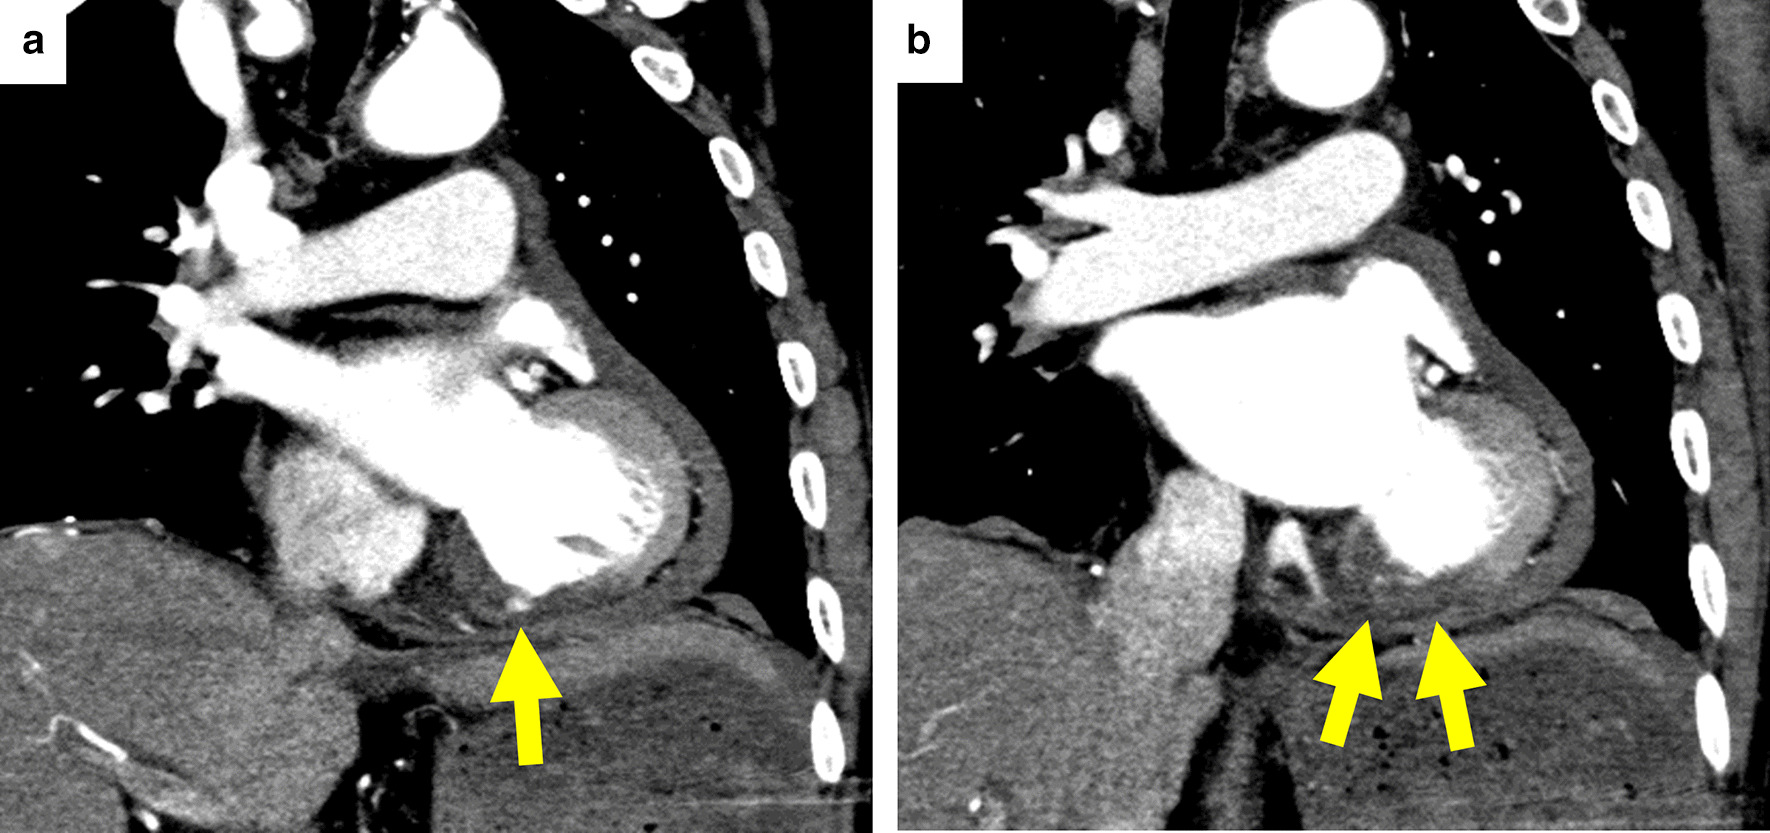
Contrast-enhanced computed tomography at the focus of left ventricle. Contrast-enhanced computed tomography shows partial protrusion of contrast medium from the inferior wall of the left ventricle (a) and poor enhancement of the inferior wall (b)
